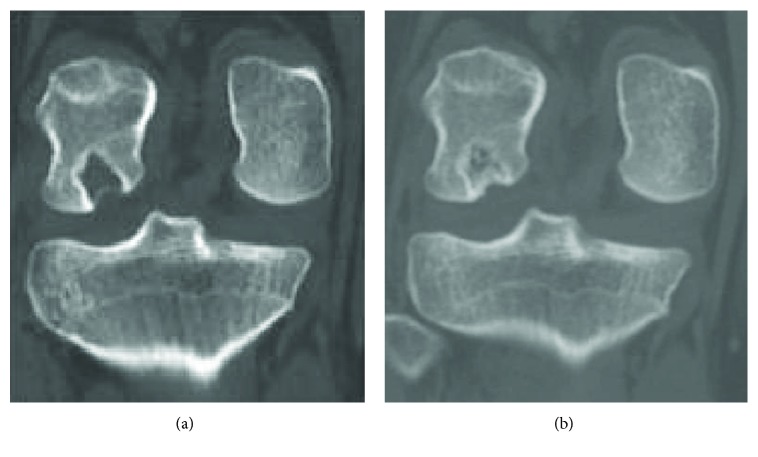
(a) Coronal computed tomography image shows a bone cavity lesion one month after the surgery. (b) Coronal computed tomography image shows the tumor bone cavity filled with bone formation one year after the surgery.
